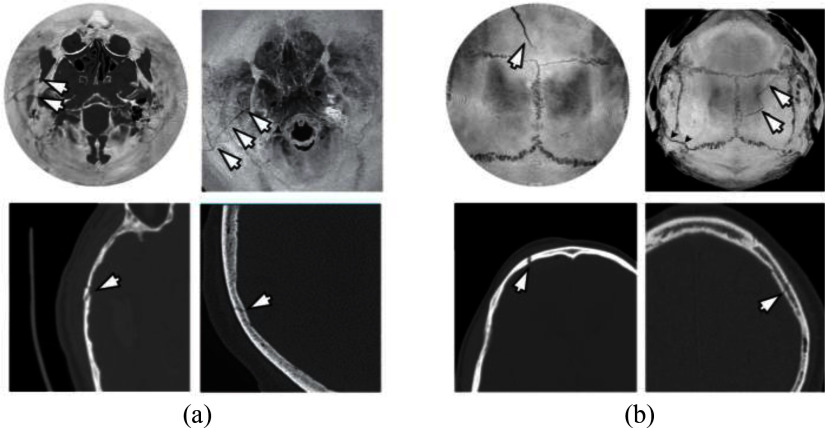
Comparison of disk harmonic mapping (left) versus skull unfold [19] (right) for visualizing (a) temporal (Patient 449) and (b) calvarial (Patient 137) fractures. Top: flattened representations. Bottom: corresponding axial CT slices magnified views of the fractured regions. Arrows indicate fracture locations. One radiologist missed the fracture in (a) whereas all 3 identified the one in (b).
